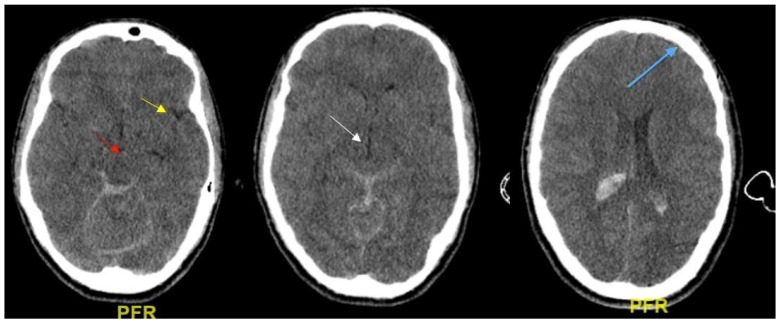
The axial CT scans evidence diffuse edema with subarachnoid and intracerebral hemorrhage: cortical sulci (blue arrow), and perimesencephalic cisterns (red arrow) are not visible, while Sylvian fissure (yellow arrow) and third ventricle (white arrow) are compressed but still visible.
